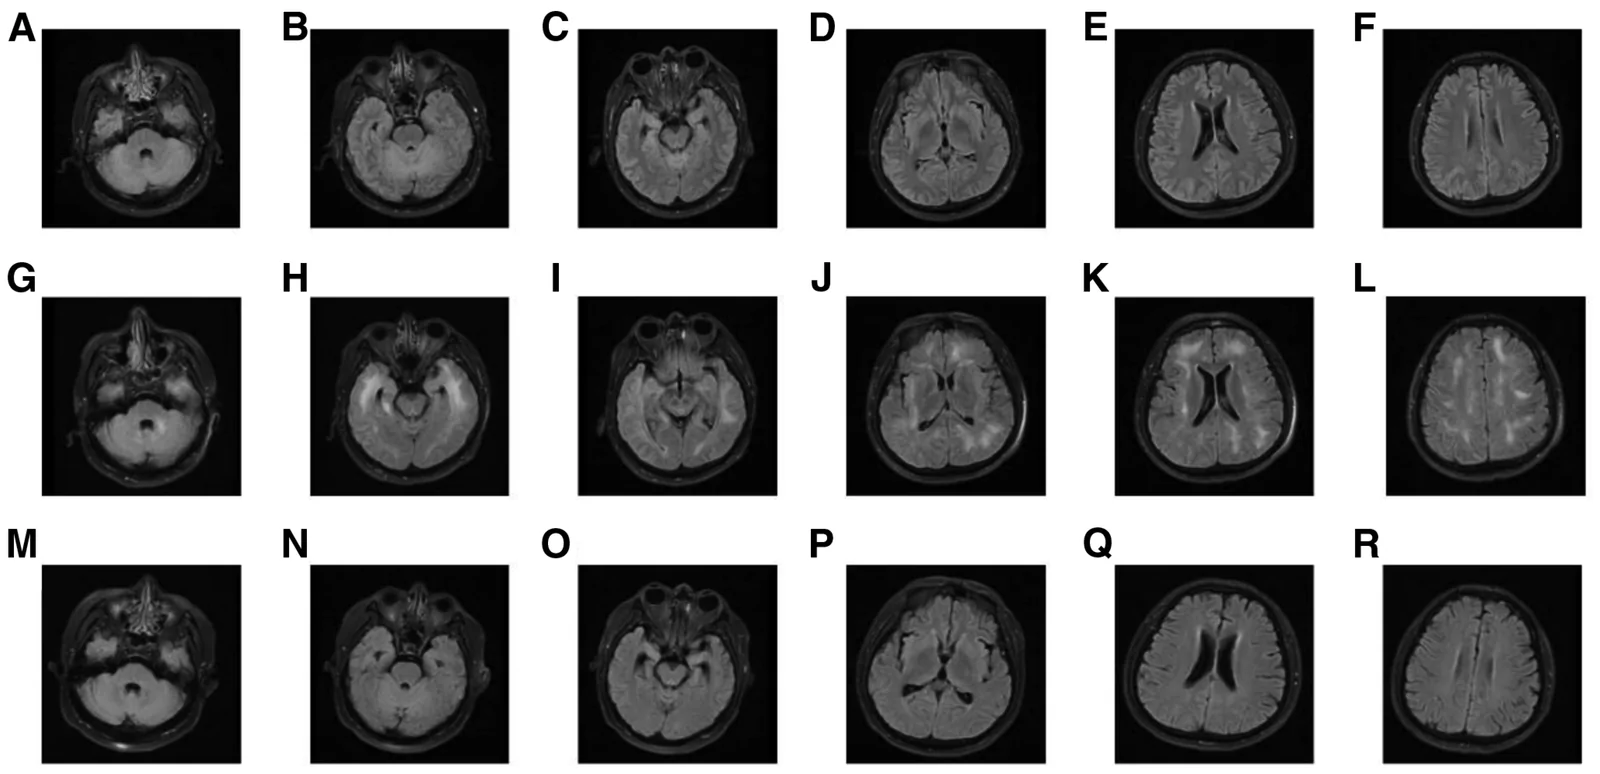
(A–F) Brain MRI showed no significant abnormal signals. (G–L) Brain MRI showed FLAIR hyperintensities in the bilateral fronto-temporo-parietal lobes, periventricular areas, insular white matter, and around the fourth ventricle. (M–R) Brain MRI showed no significant abnormal signals. FLAIR = fluid-attenuated inversion recovery, MRI = magnetic resonance imaging.
